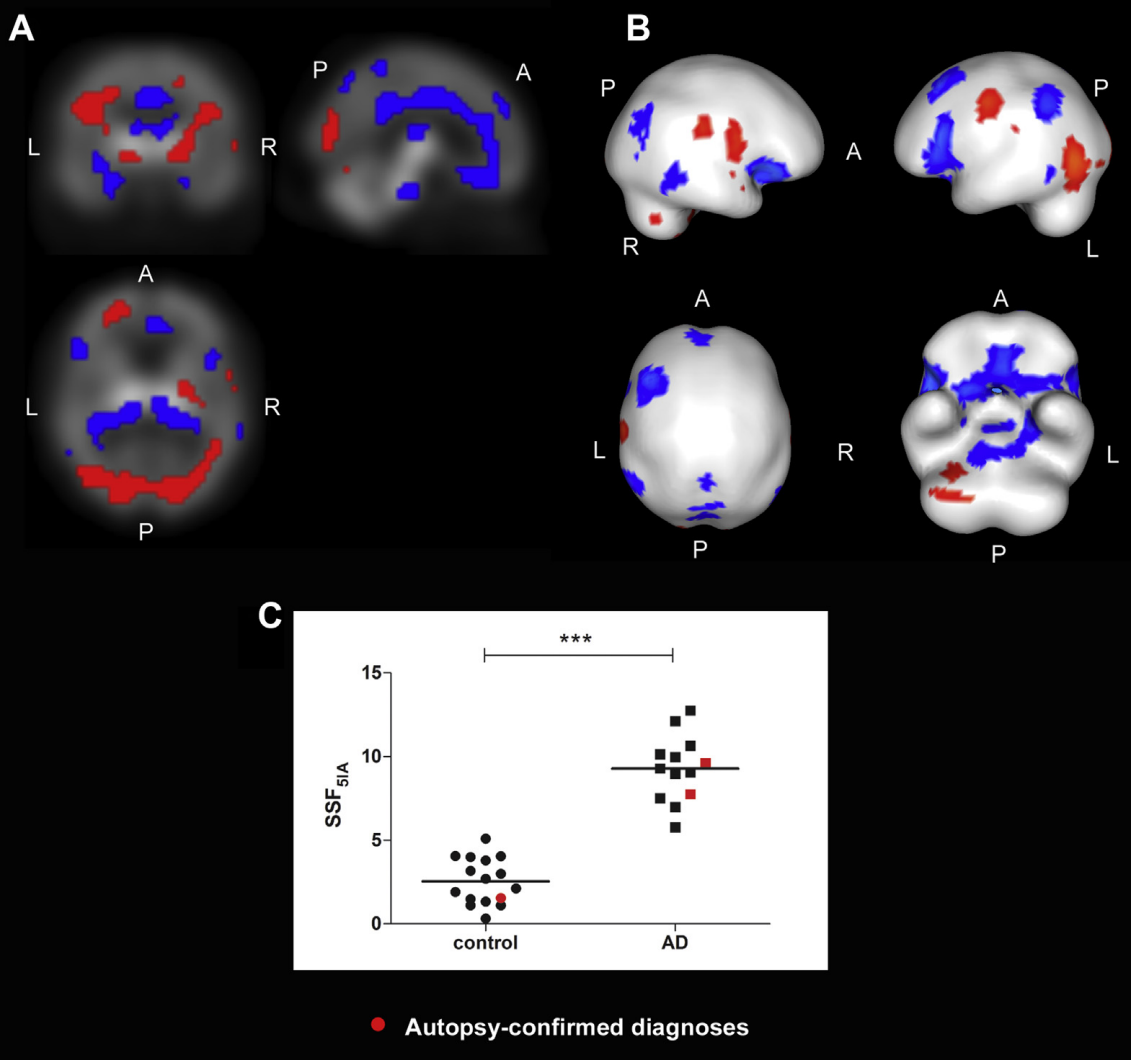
α4β2 nicotinic acetylcholine receptor spatial covariance pattern in AD (|Z| ≥ 1.64, *p* ≤ 0.05). Orthogonal (A) and rendered (B) views of the α4β2 nAChR spatial covariance pattern superimposed upon the ^12^^3^I-5IA-85380 SPECT template distinguishing AD from controls. Red and blue regions depict relative increased and/or preserved and decreased activity, respectively. (C) Distribution of SSF_5IA_ scores in controls (n = 16) and AD (n = 13) (p* < 0.001). Abbreviations: A, anterior; AD, Alzheimer's disease; L, left; nAChR, nicotinic acetylcholine receptor; P, posterior; R, right; SSF, subject scaling factor. (For interpretation of the references to color in this figure legend, the reader is referred to the Web version of this article.)
